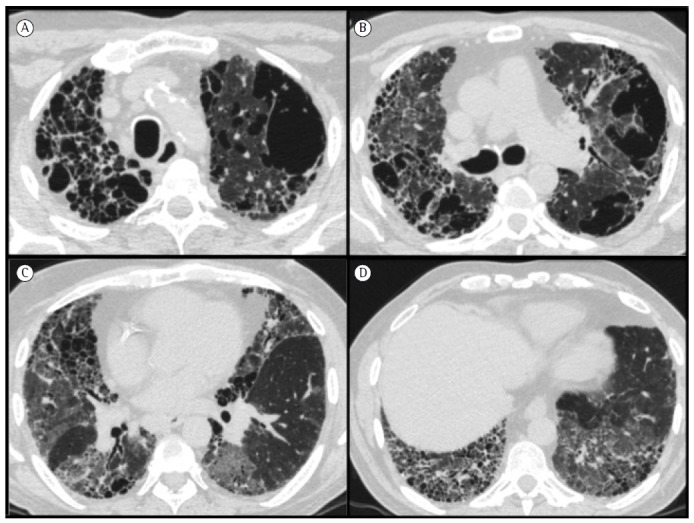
CT scan of the chest of a 70-year-old male patient with combined
